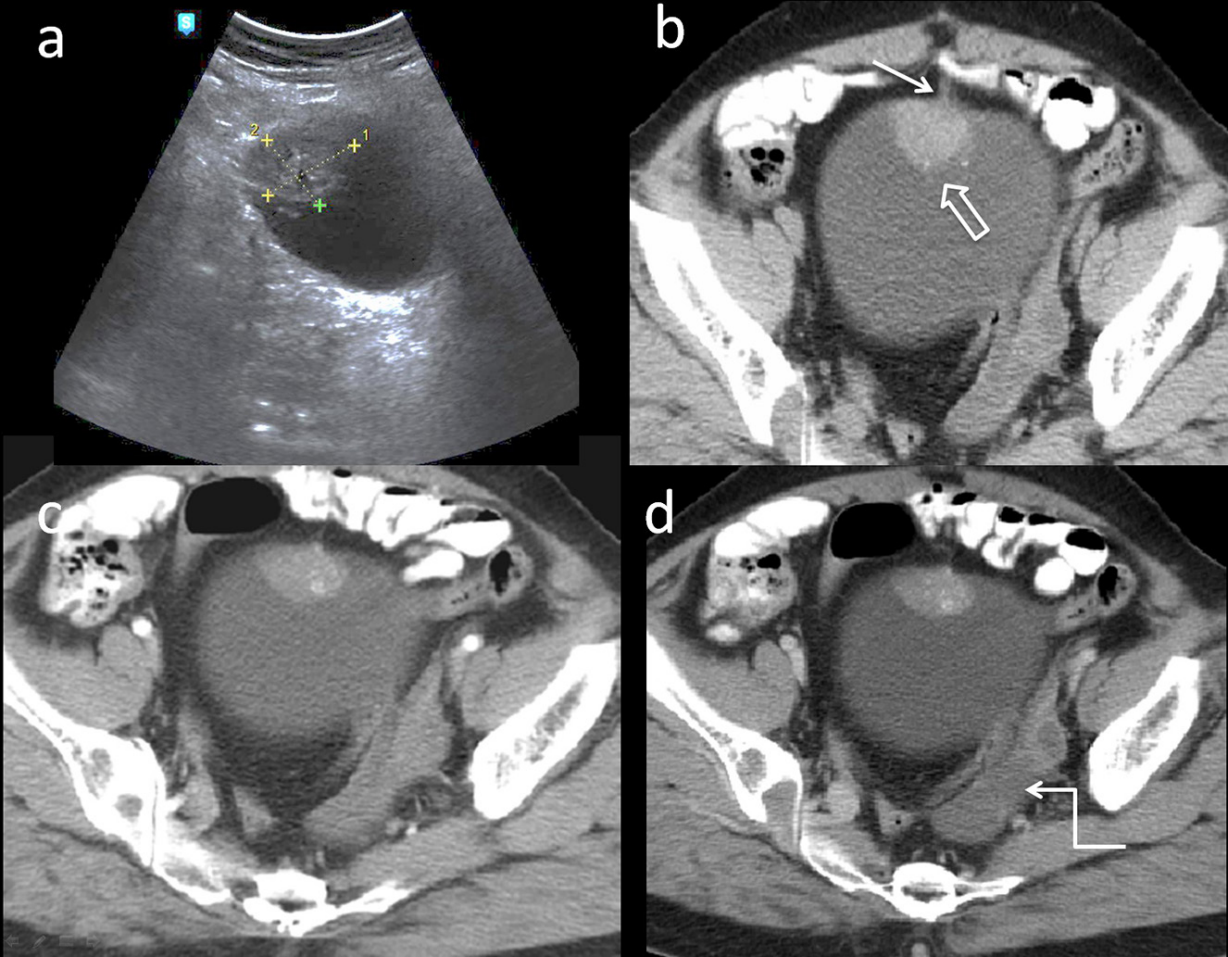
(a) 46-year-old female presented with haematuria. Sagittal ultrasound image of urinary bladder showing hypoechoic polypoidal lesion with hyperechoic foci along the anterosuperior wall of urinary bladder. Figure 1 (b) Non-contrast axial CT image of urinary bladder reveal a well-defined soft tissue density lesion with few calcific specks (open arrow) in anterosuperior wall of urinary bladder. Small, linear soft tissue density is noted between anterior abdominal wall and urinary bladder (arrow). Post-contrast axial CT images in early arterial and venous phase demonstrates heterogeneous enhancement of the lesion (c, d). Also there is left hydrosalpinx (Ziz-zag arrow).
